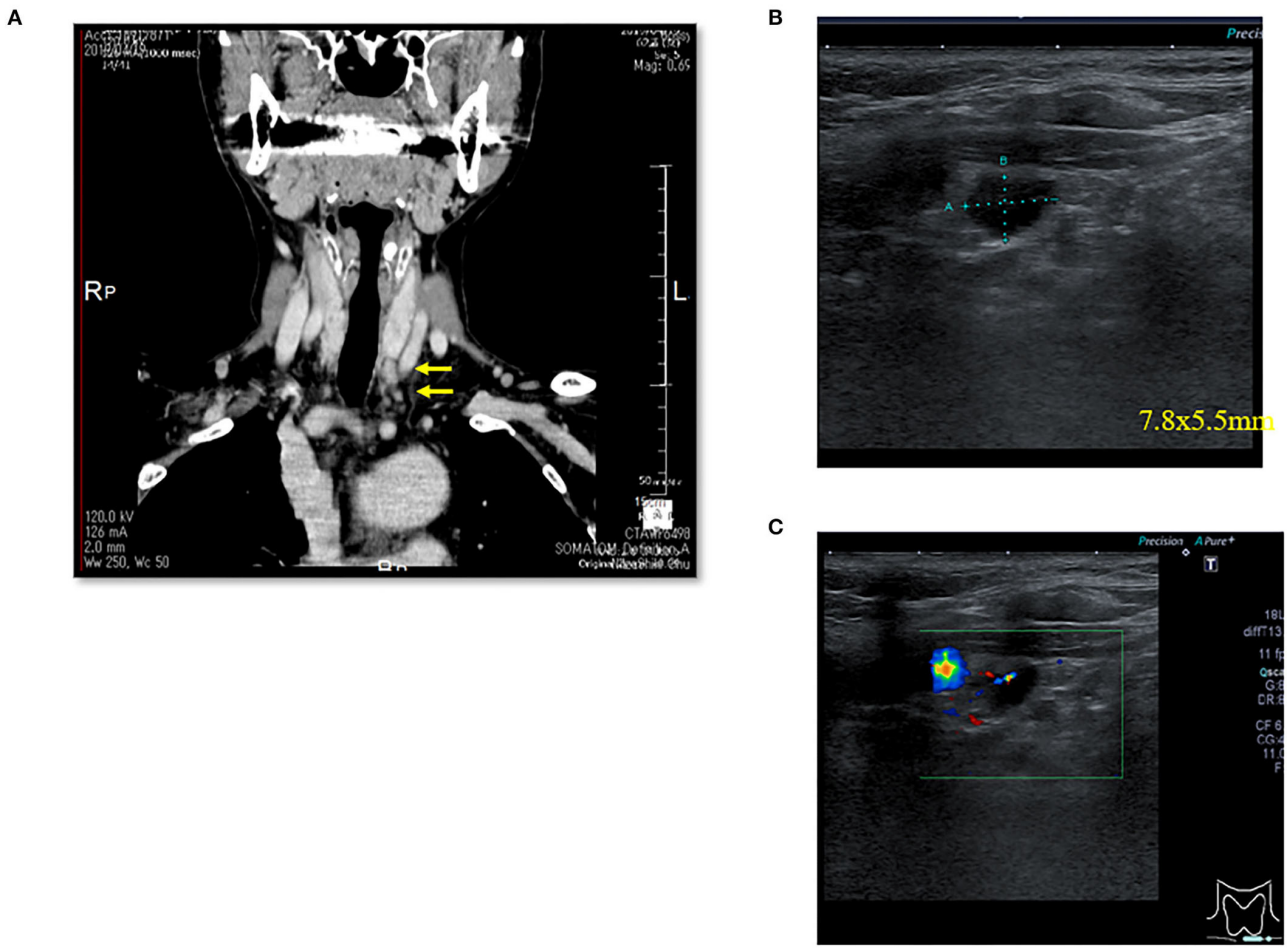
Case 4. (A) Thoracic enhanced CT (MPR) scan showing a left lower thyroid mass (EPA: yellow arrow). (B) Cervical neck ultrasound showing a left lower thyroid mass [7.8 × 5.5 mm (EPA)]. (C) Cervical neck ultrasound showing a left lower thyroid mass (EPA) and blood flow by color Doppler.
